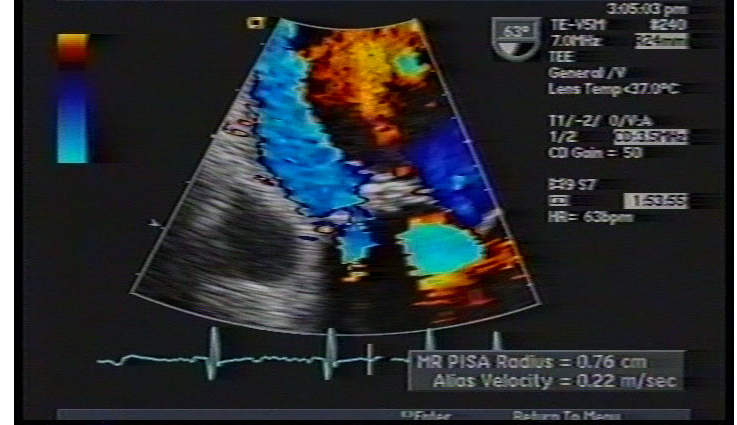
Transesophageal Echocardiogram showing (arrow) a paravalvular mitral valve leak missed by the transthoracic echocardiogram.
